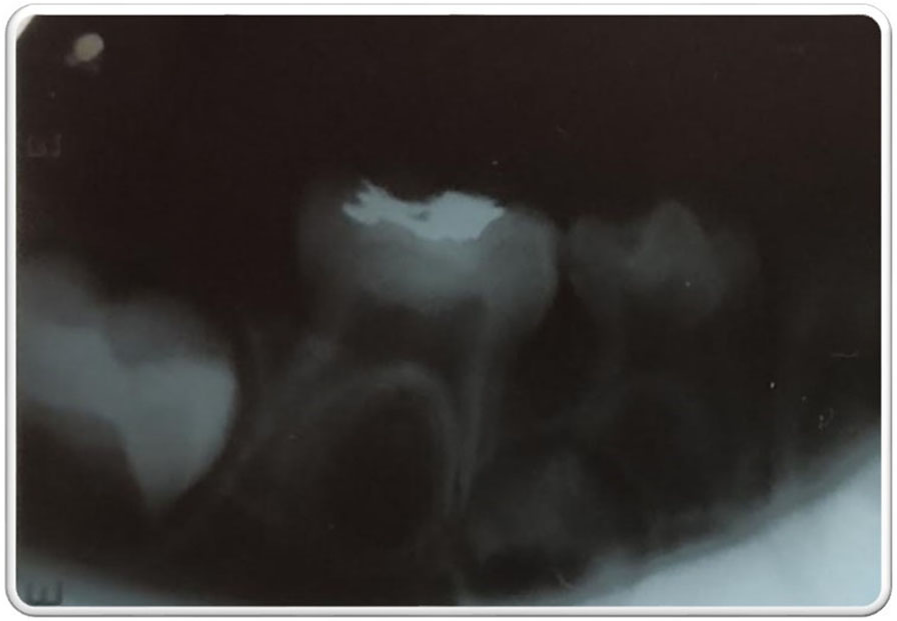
Intra-oral periapical radiograph with mandibular right posterior region showing a well-defined radiopacity on the occlusal aspect of 85. Note that the radiopacity is entirely within the enamel
